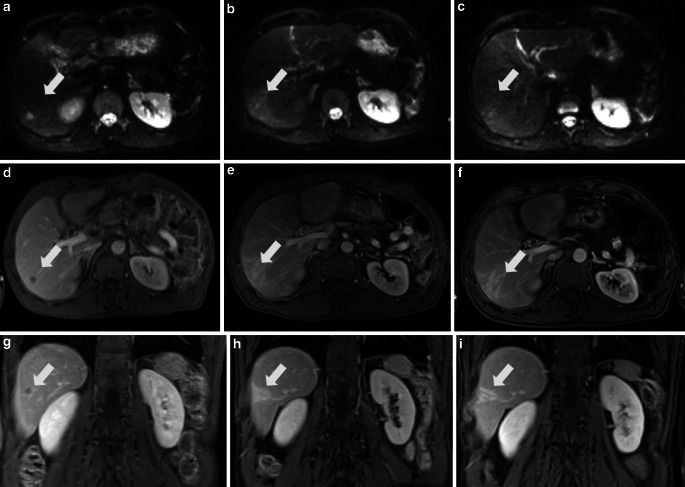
Longitudinal changes of a hepatic metastasis in the right liver lobe after stereotactic radiotherapy (SBRT). MRI sequences: diffusion-weighted imaging (DWI) transverse (a–c), contrast-enhanced T1-weighted sequence (portal-venous phase) transverse (d–f) and coronal (g–i). MRI prior to SBRT (a, d, g), 3 months after SBRT (b, e, h) and 12 months after SBRT (c, f, i). Morphological response of DWI restriction, T1‑w hypointensity after SBRT with longitudinal reduction of peritumoral changes of the normal tissue. *White arrows* highlight the region of interest including the hepatic metastasis in the right liver lobe and the peritumoral changes after SBRT
